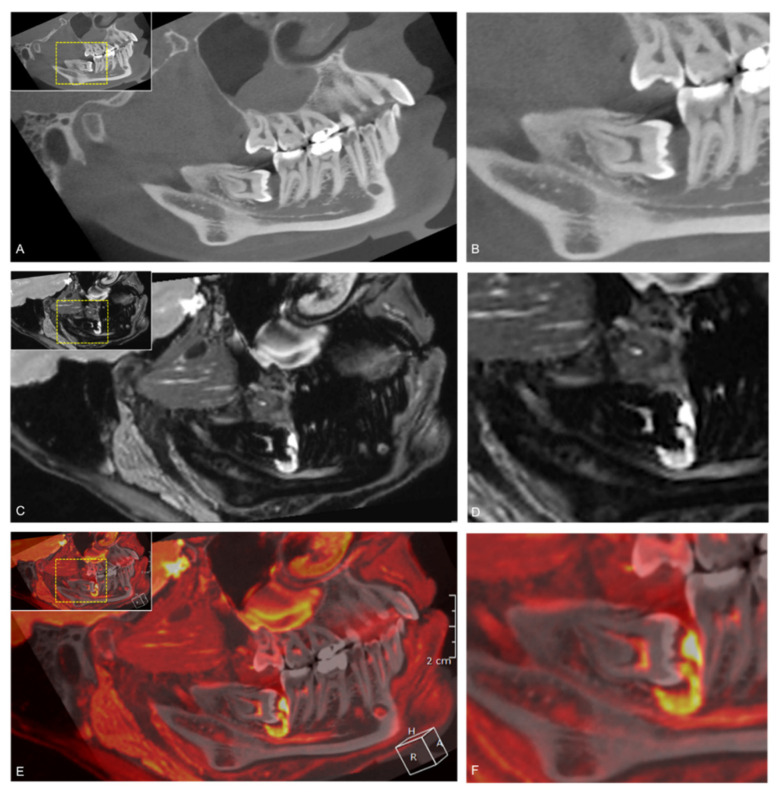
Fusion of study participant’s 3D-DESS MRI (red) and CBCT (gray). (A) Sagittal CBCT reconstruction showing an impacted mandibular third molar with mesial angulation and surrounding follicular cyst; (C) Sagittal DESS MRI visualizing an inflammatory process originating from the follicular cyst, which impressively displaces the IAN within the IAC; and (E) Fused CBCT-MRI scans visualizing the inflammatory process. For orientation, the dotted rectangles in the corner show the enlarged area in CBCT (B), MRI (D), and fused CBCT/MRI scans (F).
